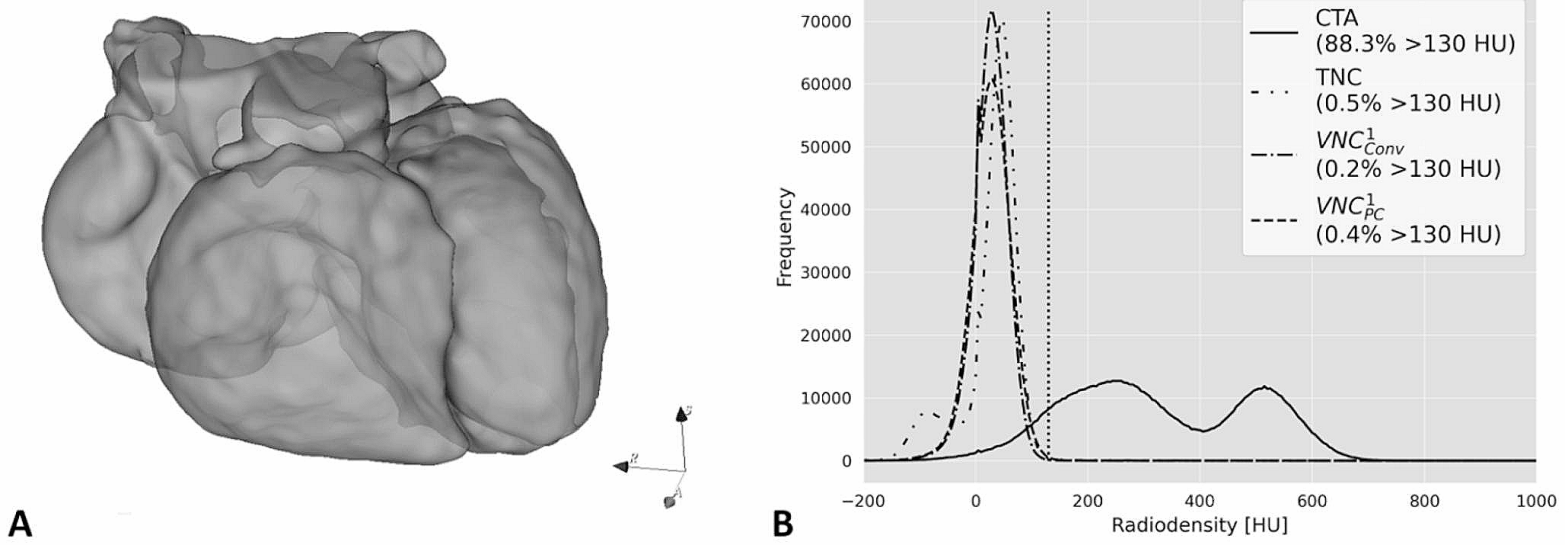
Effectiveness of iodine removal. A Demonstrates the segmentation of the whole heart and B shows the histograms based on the respective reconstruction (CTA = CT angiography, TNC = true non-contrast, VNC_conv_^1^ = conventional virtual non-contrast, and VNC_pc_^1^ = pure calcium virtual non-contrast). The histogram proportion exceeding 130 HU (marked by the dotted line) is given in the legend
